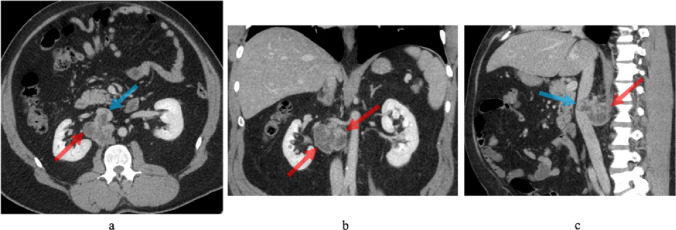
Axial (a), coronal (b) and sagittal (c) contrast-enhanced CT images through the mid-abdomen show a heterogeneously enhancing right perirenal retroperitoneal mass with a large extravascular component (red arrows) and a small intravascular IVC component (blue arrows). Pathology confirmed leiomyosarcoma arising from the IVC
